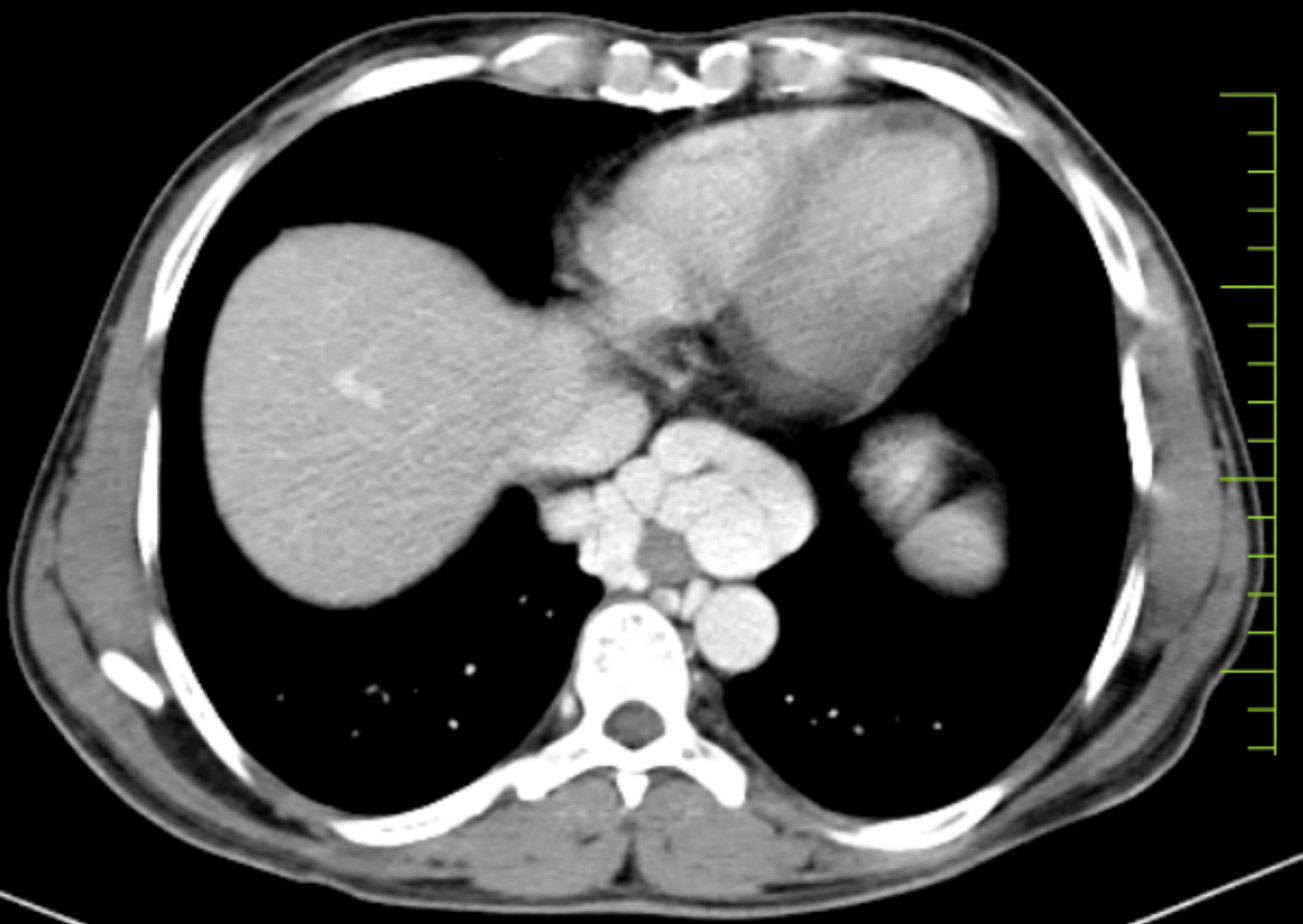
Axial CT scan image showing the expansion of esophageal varices toward the stomach
